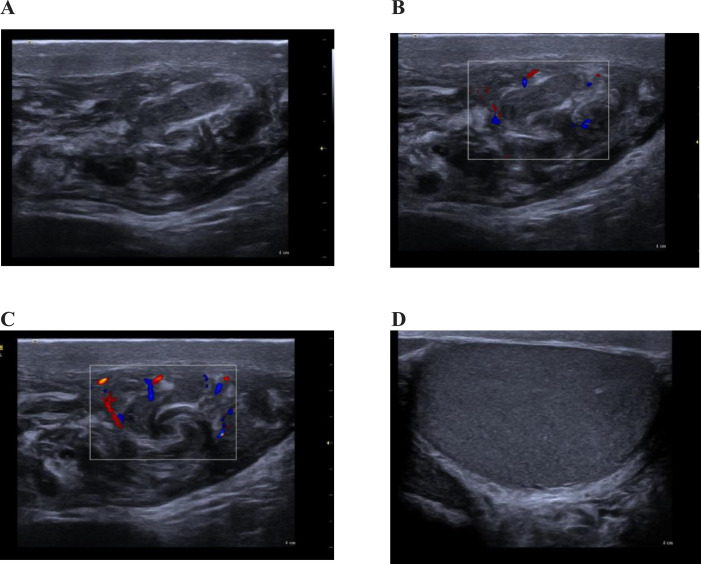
Left thrombosed varicocele (2 weeks after). (A) Shows dilated pampiniform plexuses with echogenic content. (B and C) Show no intraluminal Doppler flow noted the enlarged heterogenous epididymis due to epidedymitis. (D) Shows normal left testis with intrascrotal extension of the varicocele.
